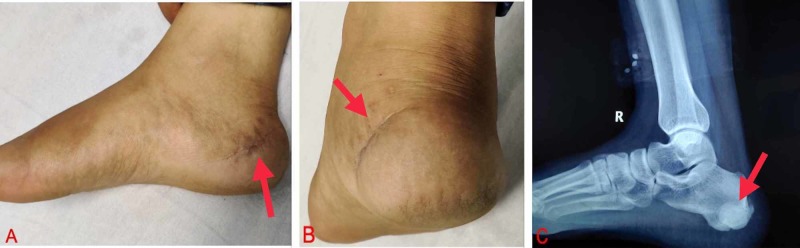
Clinical and radiographic assessment at two-year follow-up. Well-healed surgical scar with quiescent local site (A, B); X-ray having no evidence of recurrence at two-year follow-up (C).
